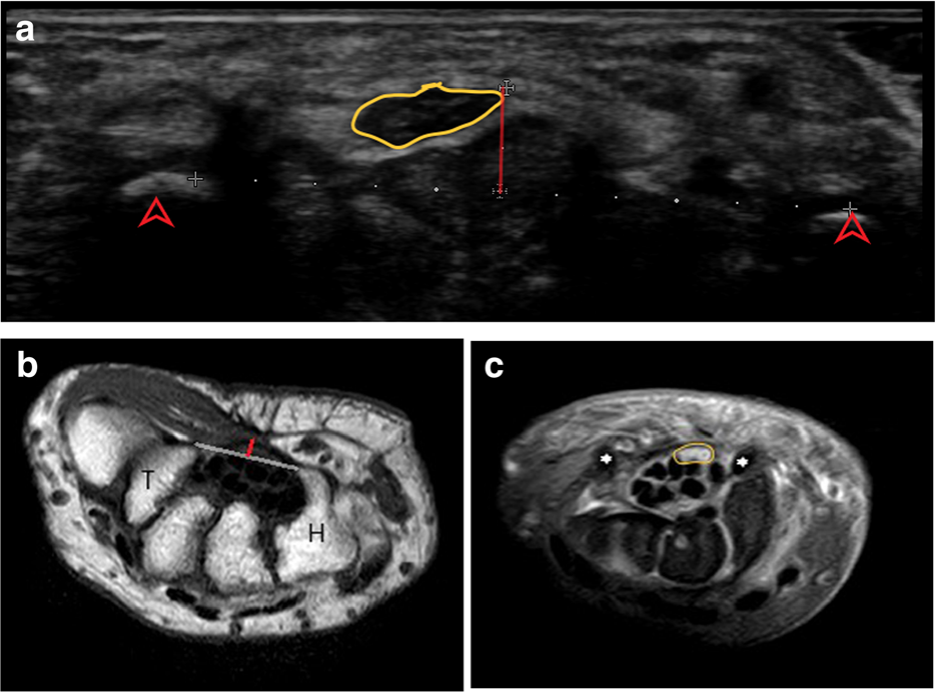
a Median nerve is hypoechoic (yellow outline) at the level of the carpal tunnel inlet (arrowhead) with bowing of the flexor retinaculum (red line) at the inlet measuring 3.4 mm in this patient with CTS. b Axial T1-weighted MR image at the carpal tunnel outlet (T- trapezium, H- hamate) shows bowing of the flexor retinaculum (red line), 2.8 mm in this patient with CTS. c Hyperintense median nerve (yellow outline) is seen on PD FS axial MR image at the carpal tunnel inlet (asterix) in the same patient with CTS
